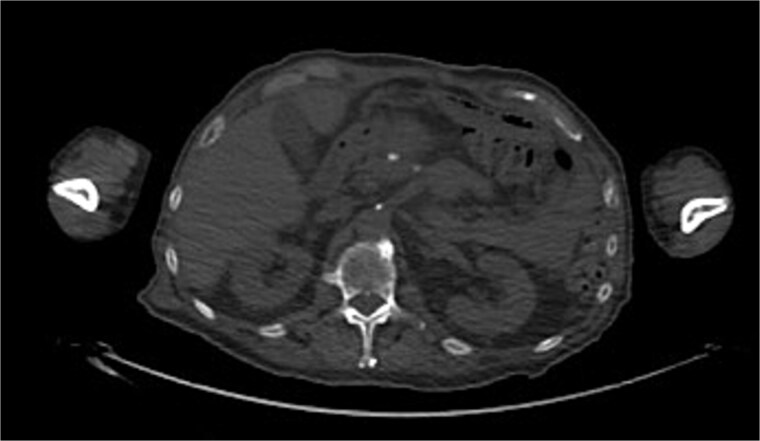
Two month surveillance CT demonstrating complete resection without recurrence.
